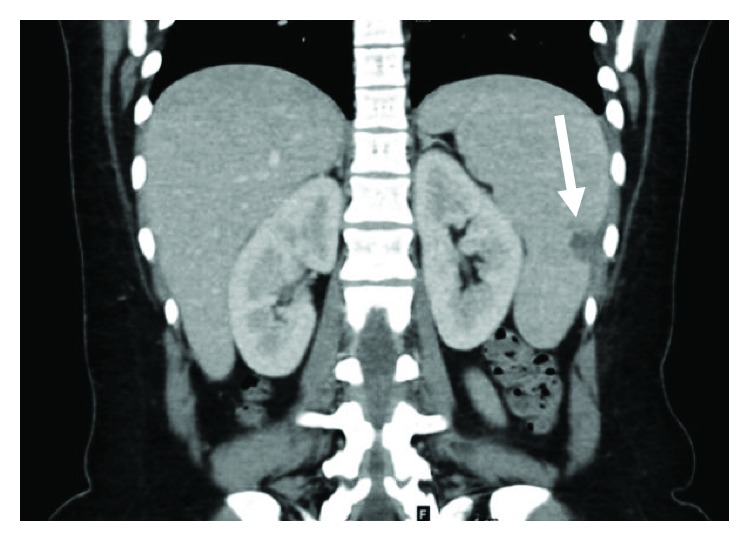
Follow-up CT scan shows hepatosplenomegaly with interval improvement regarding the splenic infarction.
